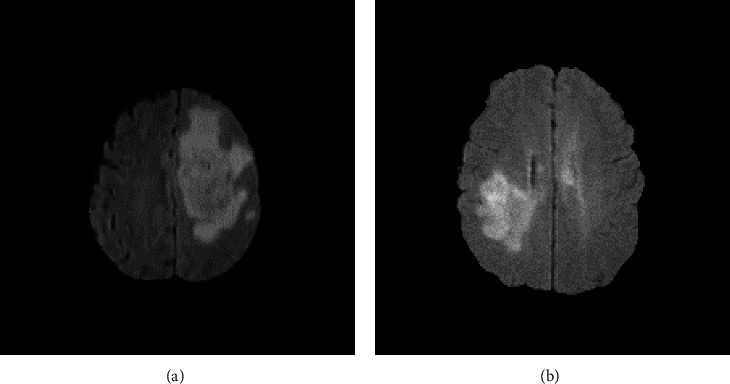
MRI with FLAIR sequence: (a) HGG; (b) LGG.
